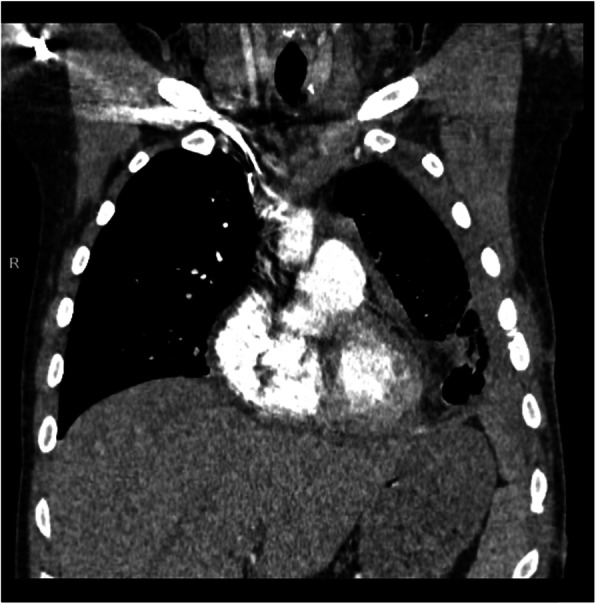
Chest CT performed 27 days after intrapericardial r-tPA treatment (mediastinal window, frontal view). Pericardial effusion, the layer of 5 mm, normal thickness of pericardium. Left sided pleural effusion with inoculations
